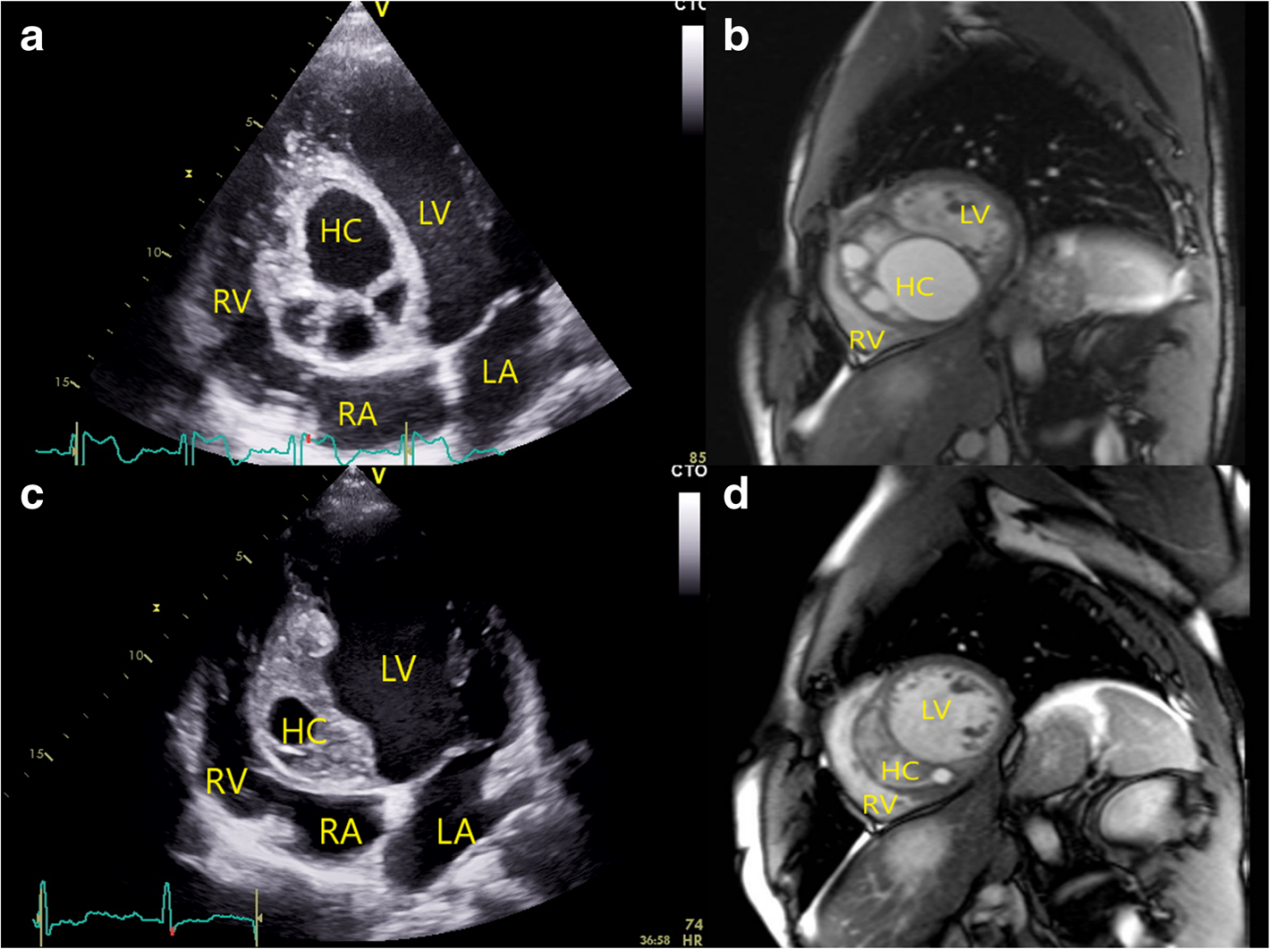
Giant hydatid cyst in the interventricular septum; (a) 2D transthoracic echocardiography, apical 4-chamber view and (b) cardiac magnetic resonance, short axis view - showing largest daughter cyst protruding in the left ventricle cavity while smaller daughter cysts protrudes in the right ventricle. c 2D transthoracic echocardiography, apical 4-chamber view and (d) cardiac magnetic resonance, short axis view – after 8 weeks of albendazole therapy, showing degradation of daughter cysts with the consequent reduction of the total hydatid cyst size. (HC = hydatid cyst, LA = left atrium, LV = left ventricle, RA = right atrium, RV = right ventricle)
